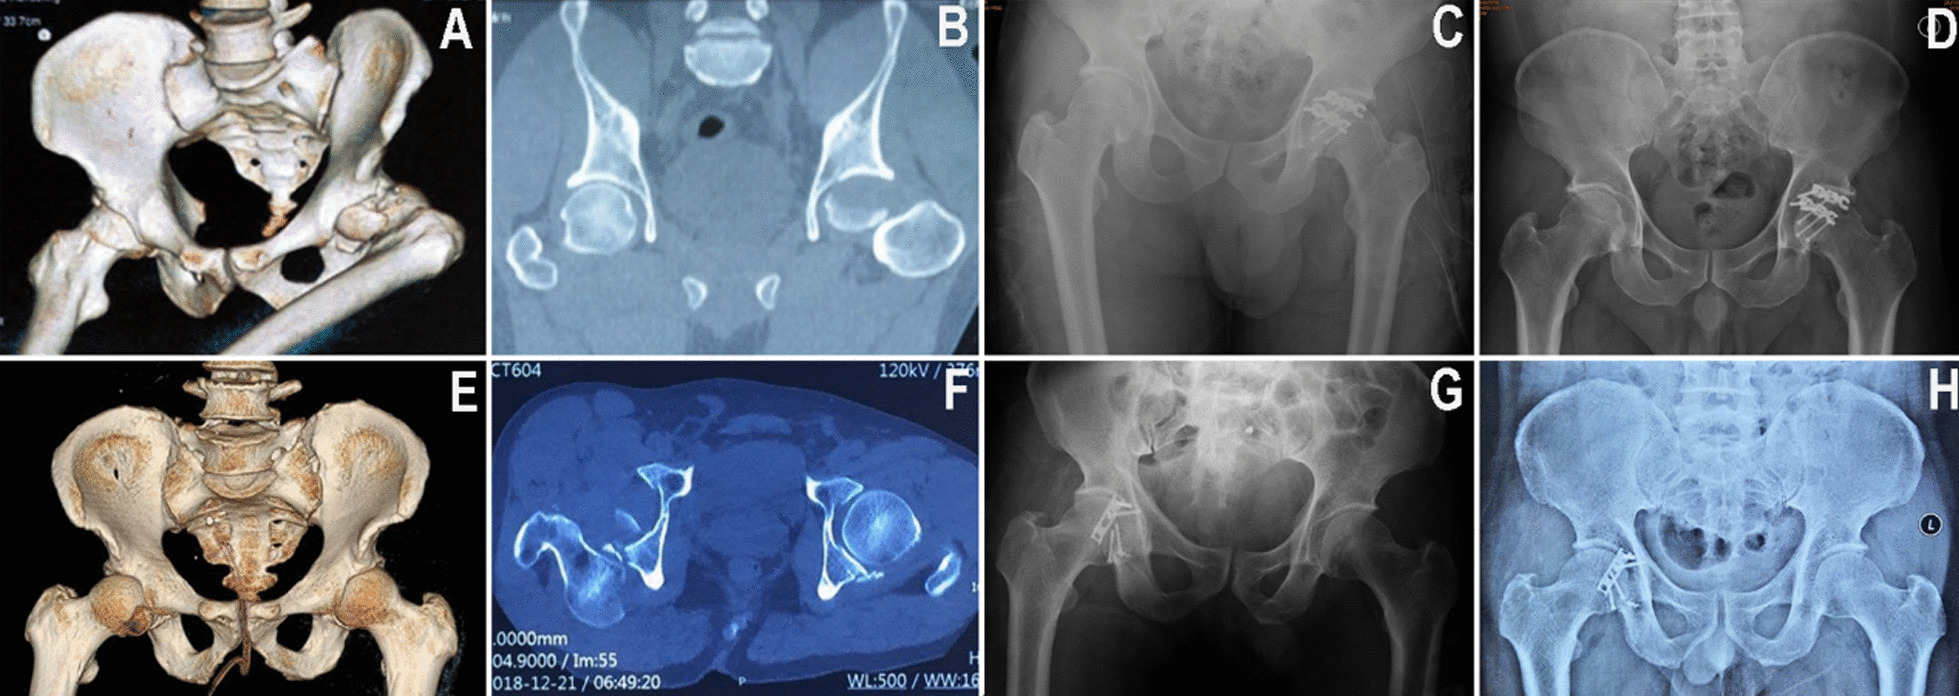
Typical case images. A, B, E, F Preoperative CT showed an acetabular posterior wall fracture and posterior dislocation of the hip. The fracture line of the left femoral head was located below the fovea. C, G X-ray examination immediately after the operation showed that the fracture was anatomically reduced, and the position of internal fixation was good. D, H X-ray examination at follow-up 1 year after operation showed that the fracture healed, no femoral head necrosis occurred, and the hip joint space was normal
